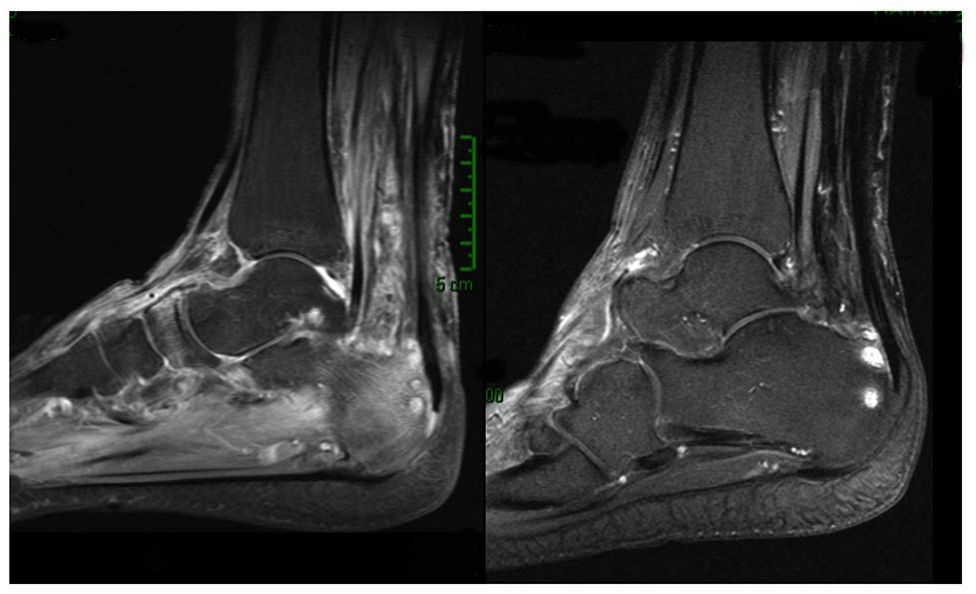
Major MRI Response in patient 2. Major MRI response in Patient 2 with disappearance of periachillean tissular infiltration and of talus bone edema. Slight talus contrast enhancement persistance on T1 Gado Fat Sat sequence.
